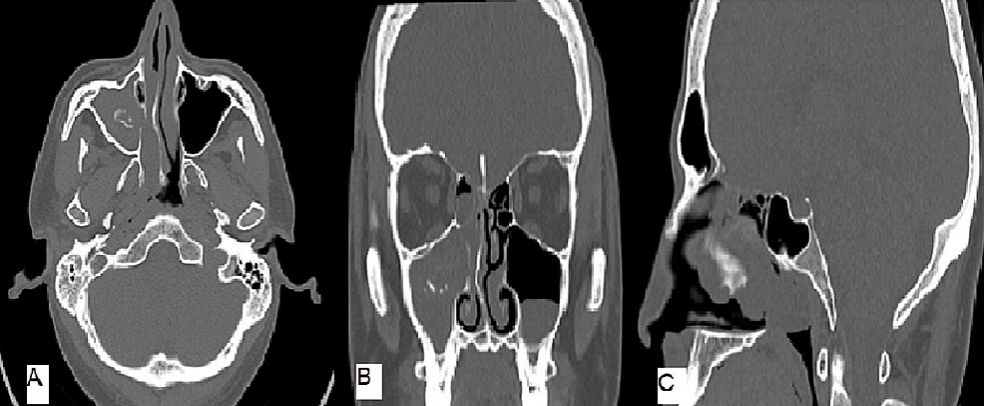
Computed tomography images of paranasal sinuses, showing soft tissue opacification within the right maxillary sinus with extension into the nasal cavity and choana along with the central area of calcific density seen within the right maxillary sinus, A: Sagital view, B: Coronal view, C: Axial view.
